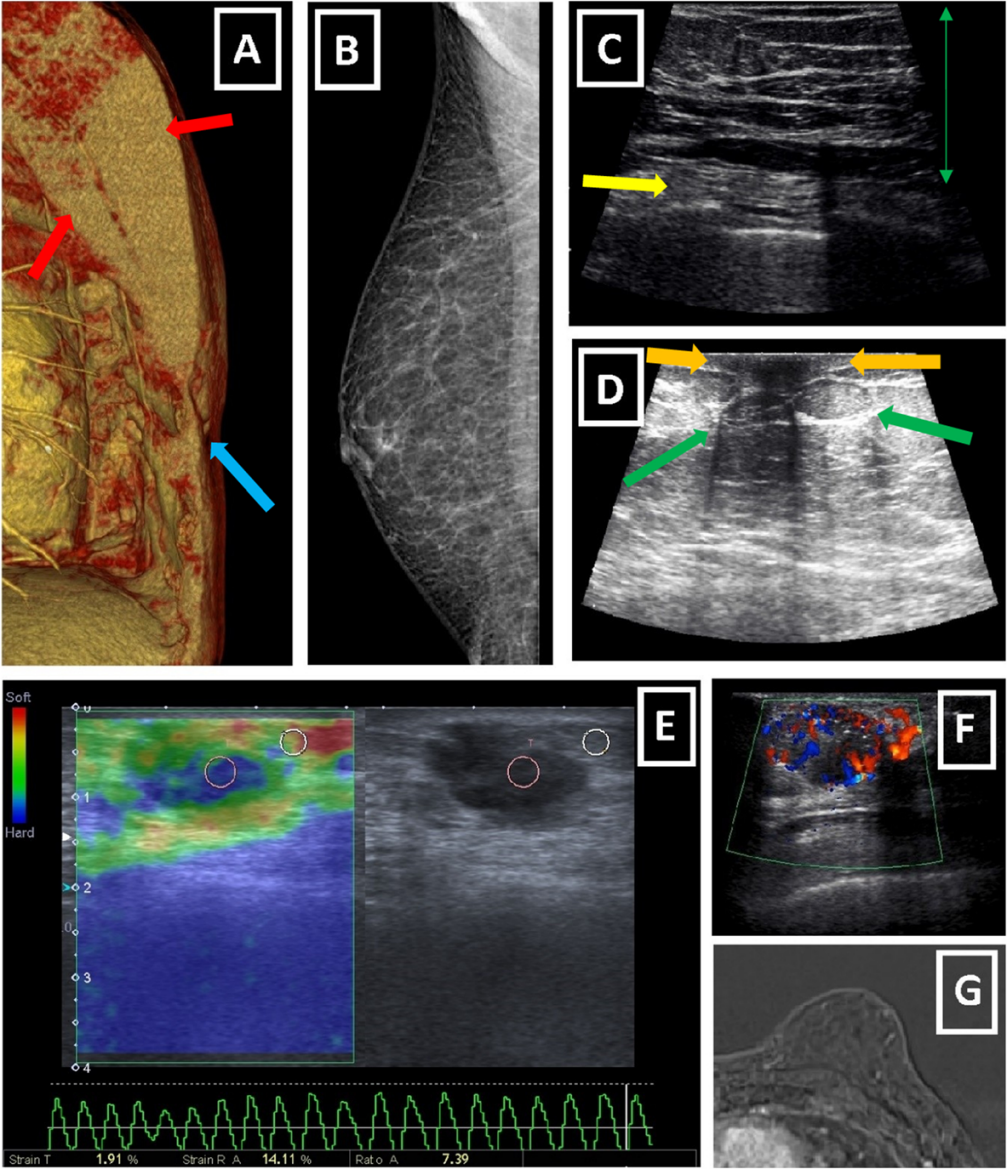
Imaging modalities used for male breast diseases. a Oblique sagittal VRT reformat of a CT scan obtained for an irrelevant purpose shows hypertrophic pectoralis major and minor muscles (red arrows) and retroareolar scarce fat tissue (blue arrow). Both can cause technical and diagnostic difficulties for mammography. Although it can show masses incidentally, CT is not a routine imaging modality for male breast. b MLO mammogram of right male breast shows rudimentary fibroglandular tissue replaced with increased amount of radiolucent adipose tissue. c, d US images of male breast. Green arrows show subcutaneous fat tissue characterized by isoechoic fat lobules. Yellow arrow (c) represents intercostal muscles and orange arrow (d) corresponds to small nipple-areola complex with no associated fibroglandular tissue. e Sonoelastographic evaluation of ill-defined hypoechoic male breast mass reveals elevated tissue stiffness with considerably increased strain ratio of 7.39 and suggests malignity. Pathologic examination confirms the diagnosis of IDC. f Color mode of Doppler US shows markedly increased peripheral and internal vascularity of irregular hypoechoic male breast mass which is diagnosed as IDC later. g Subtraction image of dynamic breast MRI of a male patient with known pseudogynecomastia shows enlarged left male breast without apparent enhancing lesion
